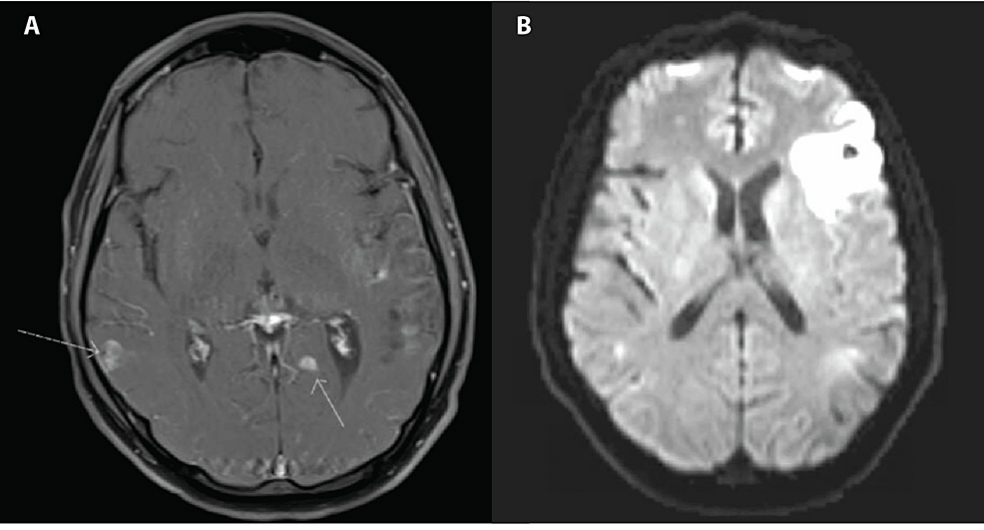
MRI and MRA findings of diffuse focal lesions and infarction A. Nodular foci of enhancement on MRI on T1-weighted image in the axial plane indicated by arrows concerning metastasis, infection, or vasculitis lesions. B. New area of infarct in the left frontal region on MRA on T2-weighted image in the axial plane. MRA: magnetic resonance angiogram.
